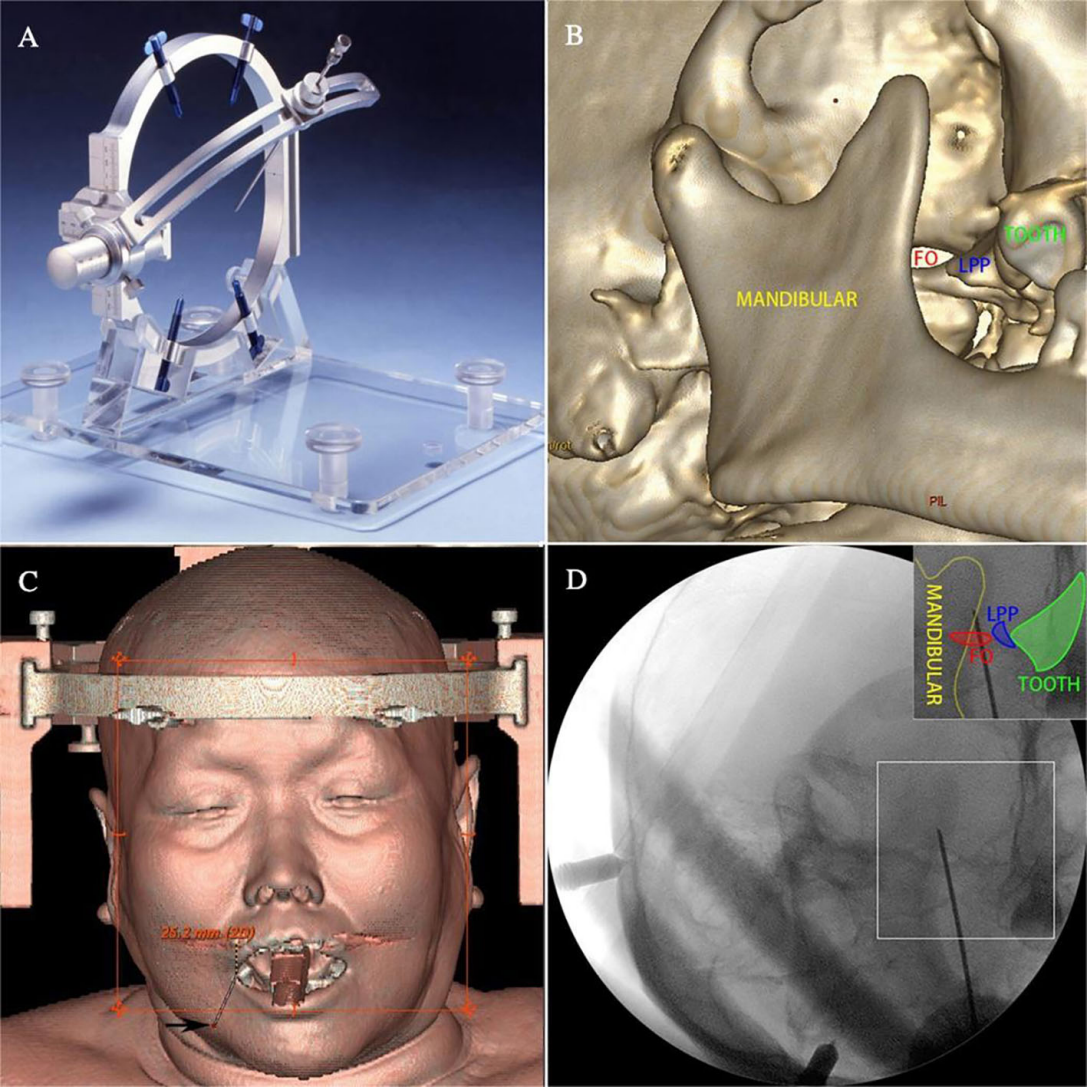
PSR surgical equipment and procedure. (A) Komai’s frame-based CT-Stereotactic system. (B) 3D CT reconstruction image for FOT localization. (C) Application of the air-to-air meeting technique to complete the puncture. (D) X-ray C-arm verification of the puncture needle reaching the FOT.
